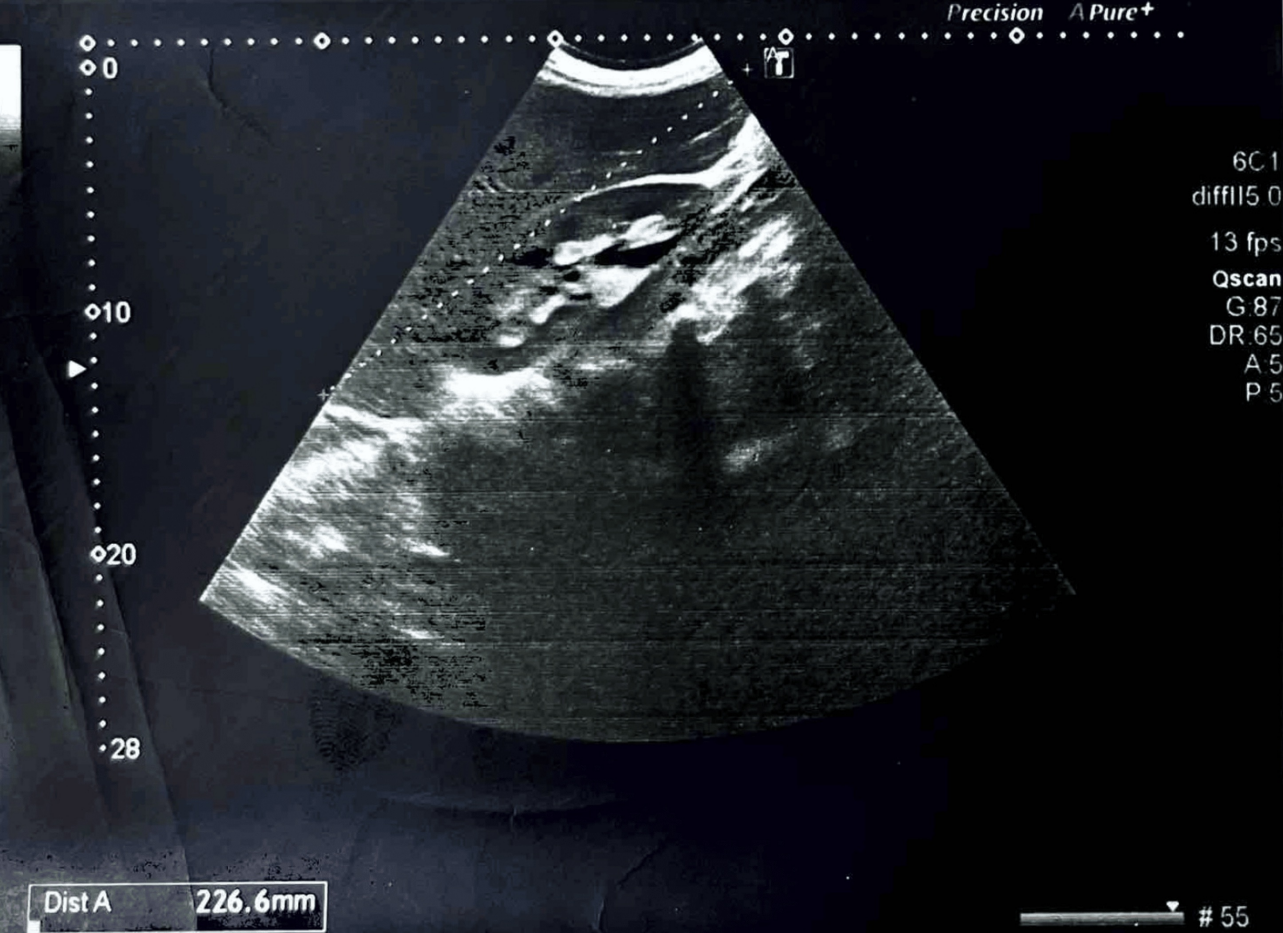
Ultrasound of the abdomen.
